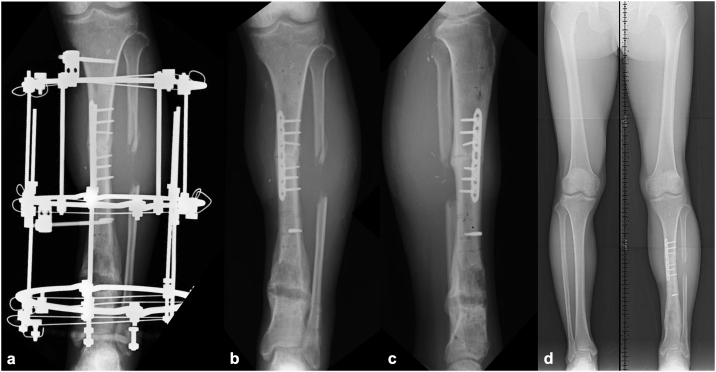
Radiographic findings after the limb lengthening. (a) Anteroposterior radiograph at 94 days after the application of the Ilizarov external fixator (IEF). (b) Anteroposterior and (c) lateral radiographs at 196 days (post-IEF removal). (d) Weight-bearing anteroposterior radiograph of both lower limbs 6 months post-injury showing no evident leg-length discrepancy.
